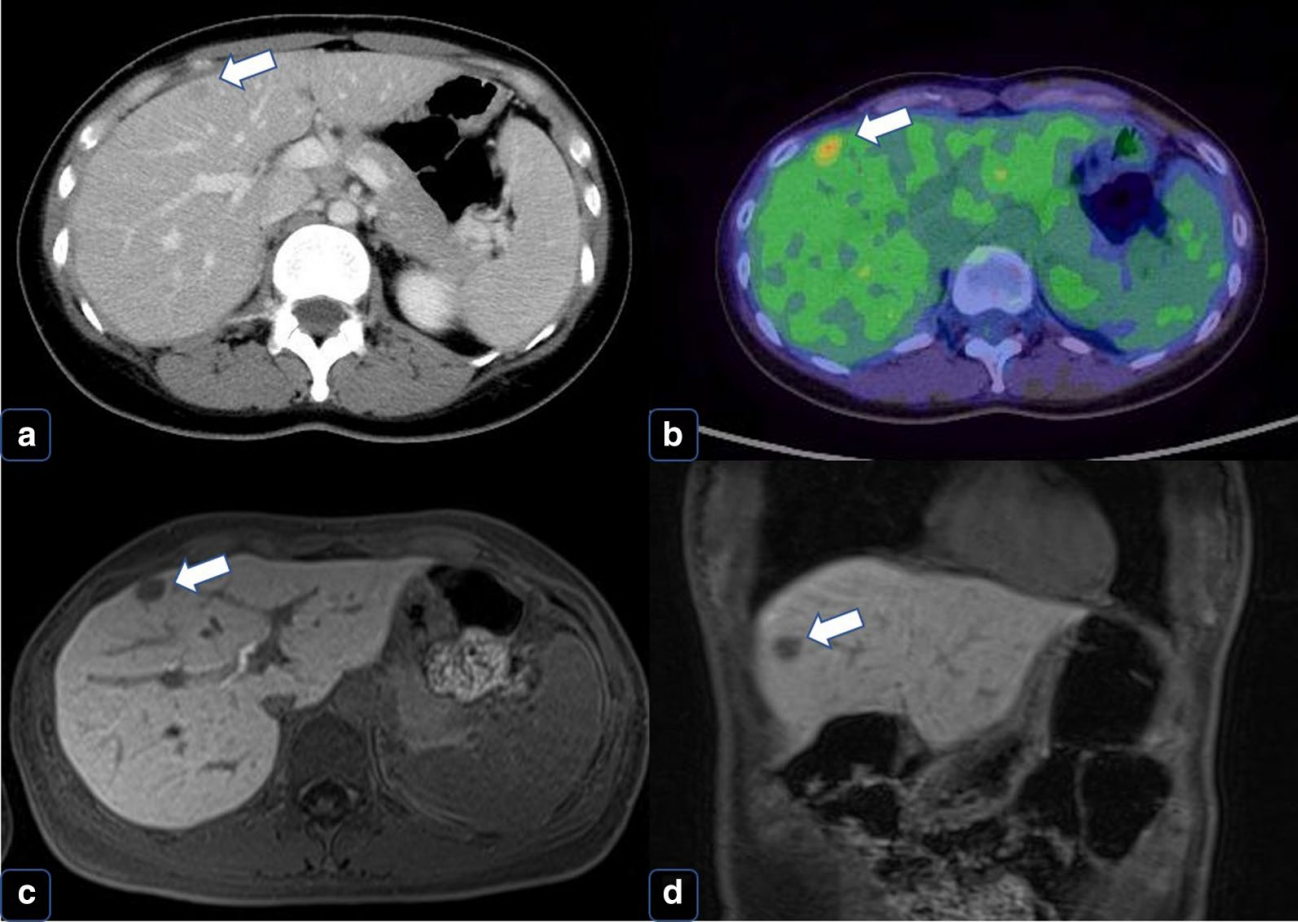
Findings at a single liver metastasis. a CT. Horizontal view. Liver metastasis (the white arrow). b PET (the white arrow). c EOB-MRI. Horizontal view (the white arrow). d EOB-MRI. Sagittal view (the white arrow)
